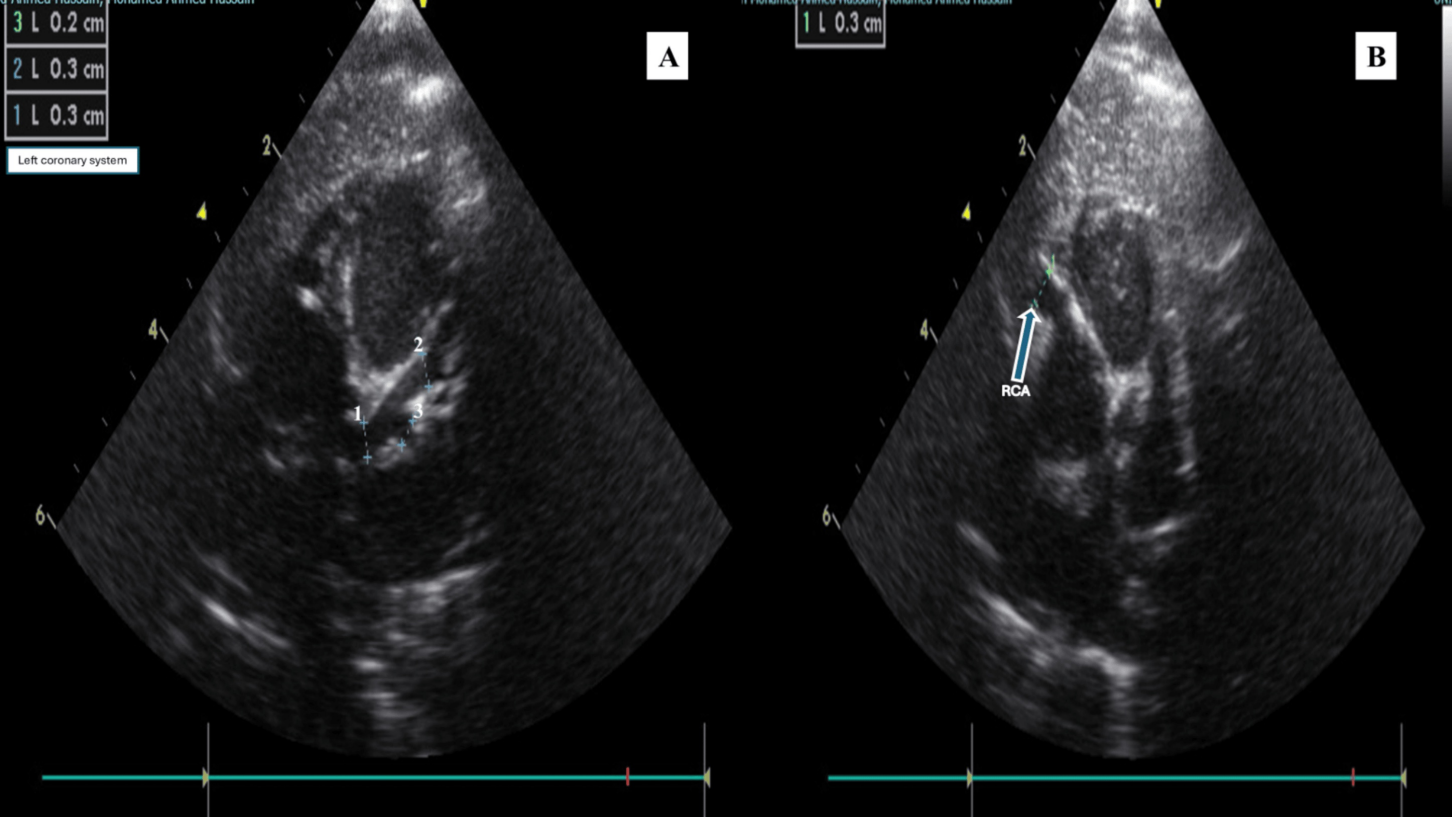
Coronary artery aneurysms at diagnosis on transthoracic echocardiography (A) Left coronary system showing coronary dilation. The on-screen caliper measurements correspond to: 1 = left main coronary artery, 2 = left anterior descending artery, 3 = left circumflex artery. (B) Dilated right coronary artery (arrow); on-screen calipers indicate the measured coronary artery diameter
